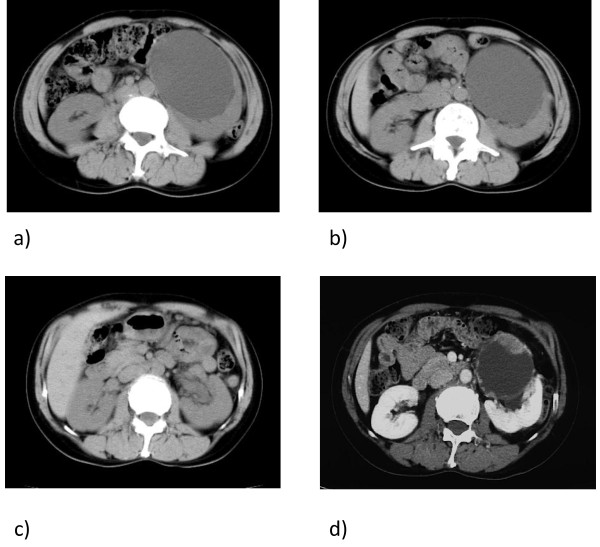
(a) Abdominal CT acquired in May 2005.CT images revealed a renal cyst approximately 9 cm in diameter in the middle of the left kidney. Renal cyst sclerotherapy with minocycline was performed in May 2005. (b) Abdominal CT acquired in November 2005. After sclerotherapy with minocycline, the renal cyst regrew to its original size within 6 months. Therefore, sclerotherapy with 95 % ethanol was administered. (c) Abdominal CT from July 2007. The CT images showed no regrowth of the renal cyst nearly two years after second-line ethanol sclerotherapy. (d) Enhanced abdominal CT from January 2009 revealed a cystic renal mass approximately 7 cm in diameter containing an irregular enhanced mass lesion.
